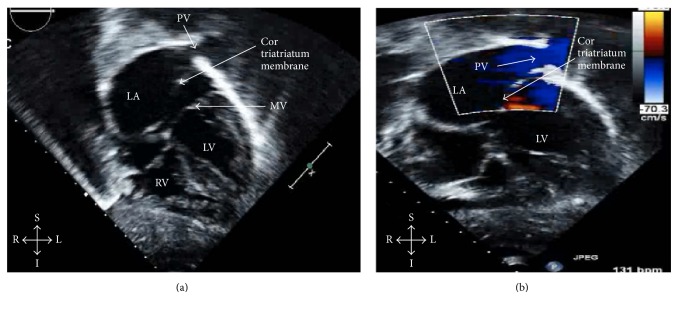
Subcostal coronal view on echocardiogram (a) demonstrates cor triatriatum with a membrane separating the pulmonary veins from the left ventricle inflow and (b) is a Doppler image demonstrating restricted flow through the opening of the cor triatriatum membrane with high-velocity flow through a dilated left pulmonary vein. LV: left ventricle, RV: right ventricle, LA: left atrium, RA: right atrium, MV: mitral valve, PV: pulmonary vein, S: superior, I: inferior, R: right, and L: left.
